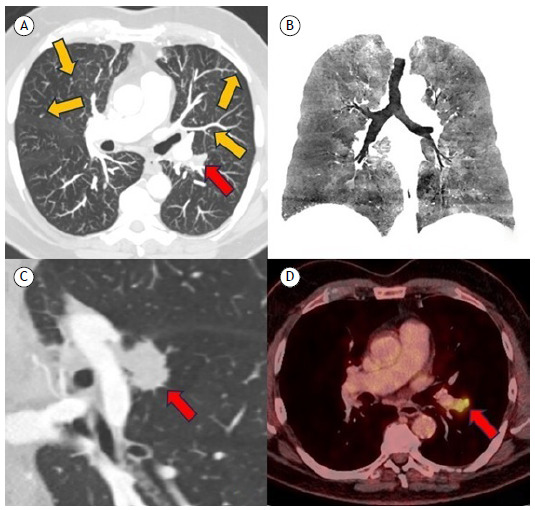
A: an axial CT image in lung window setting reformatted in maximum intensity projection depicts multiple lung nodules (yellow arrows) in line with the diagnosis of diffuse idiopathic pulmonary neuroendocrine cell hyperplasia (DIPNECH); the main lesion is shown in A (red arrow). In B, a coronal CT image in lung window setting reformatted in minimal intensity projection demonstrates mosaic attenuation in the lungs, commonly found in DIPNECH. In C, a coronal CT image in lung window setting shows the morphological characteristic of irregular margins of the main lesion (red arrow), which distinguishes it from the other lesions. In D, a fusion PET-CT image demonstrates focal glycolytic hypermetabolism in the lesion (red arrow); none of the other smaller nodules showed significant ^18^F-fluorodeoxyglucose uptake.
